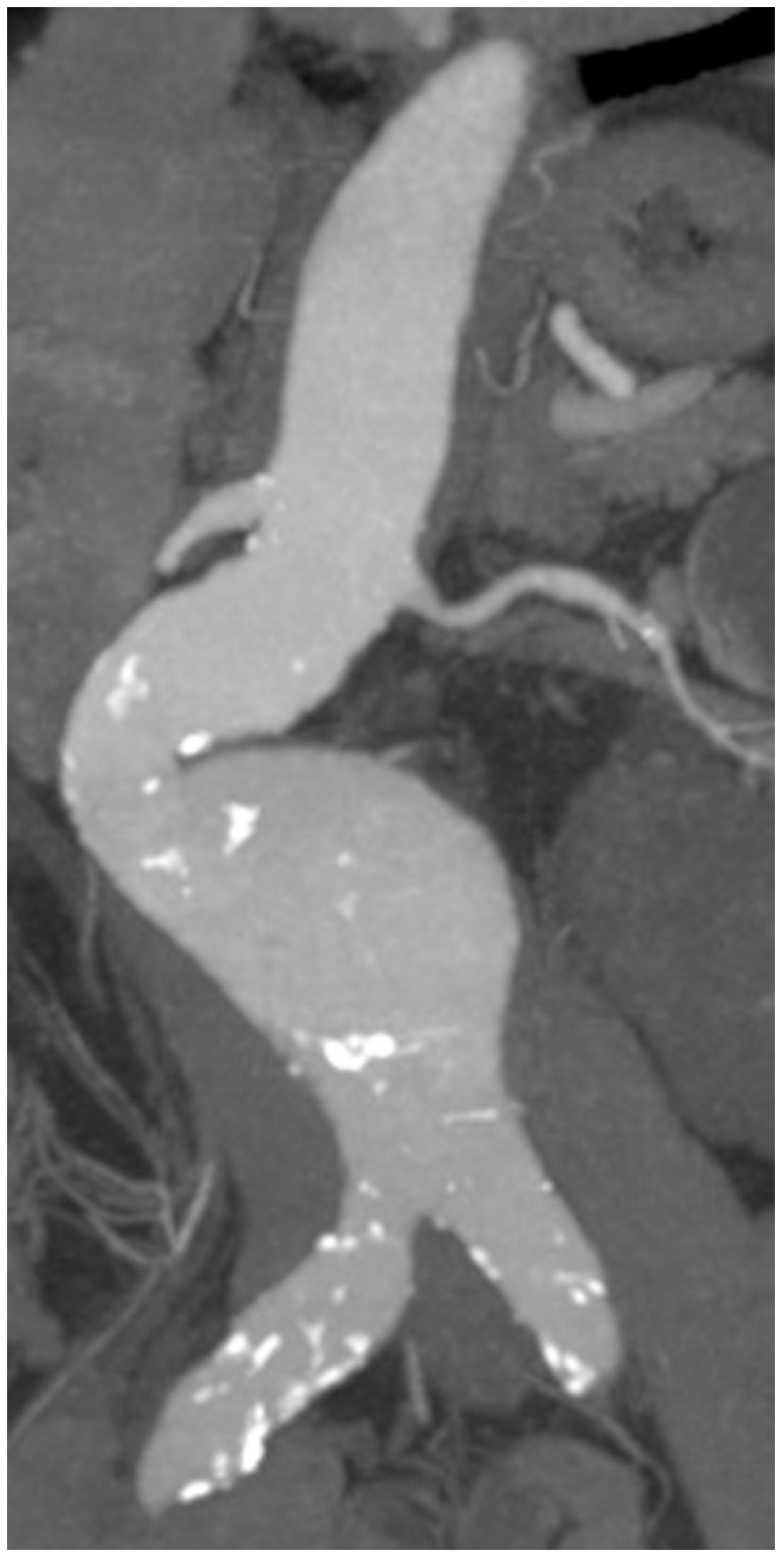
CT recording of an infrarenal AAA with eccentric shape and sites of calcification (atherosclerotic plaques highlighted in white).
